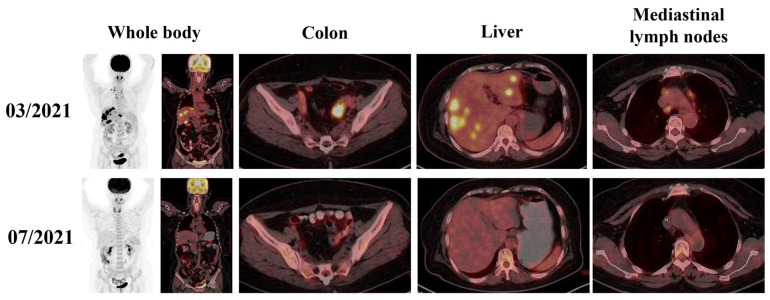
PET/CT scans show the changes in metabolic activity of the whole body, colon, and liver metastases of the patient. In the baseline time (March 2021), PET/CT scans revealed a colorectal tumor (SUVmax, 16.4; SUVave, 10.2), multiple liver metastases (SUVmax, 23.9; SUVave, 13.9), and multiple lymph node metastases (SUVmax, 7.5; SUVave, 4.5). After first-line chemotherapy and three courses of combination treatment, a complete metabolic response was achieved.
